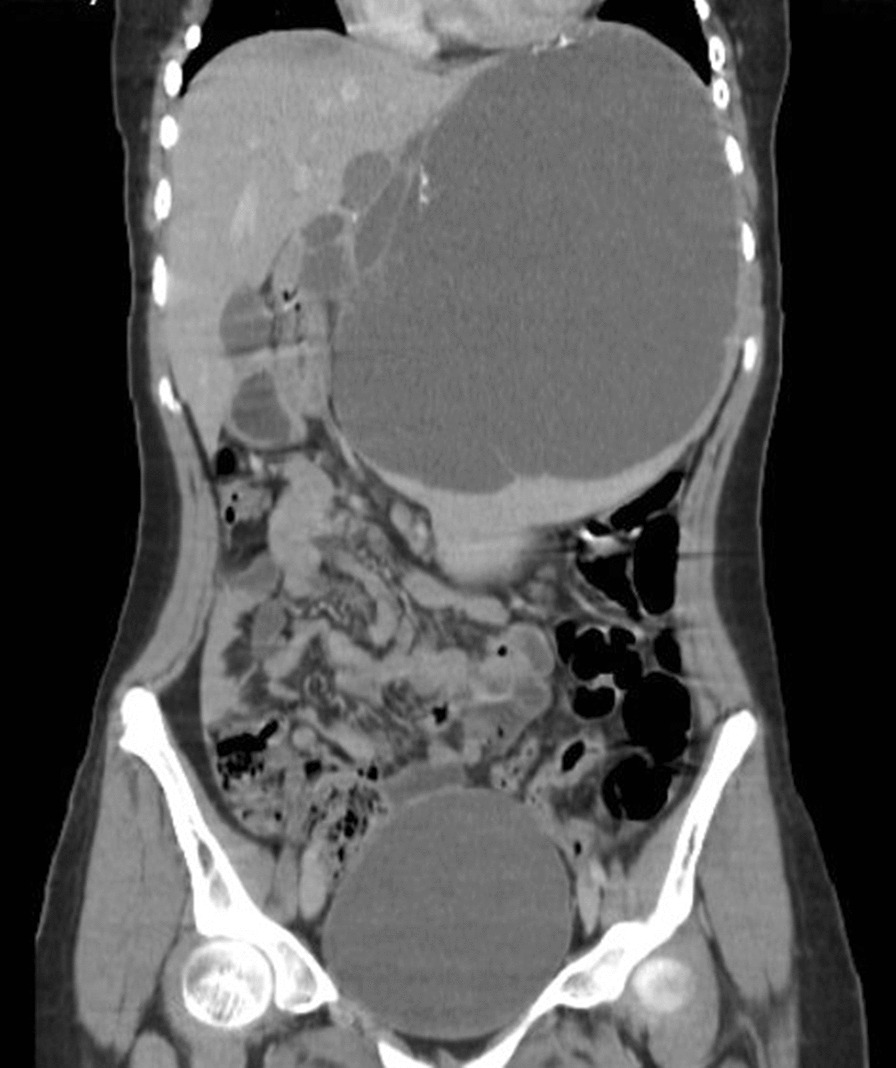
Computed tomography scan revealing a giant cyst in the spleen
